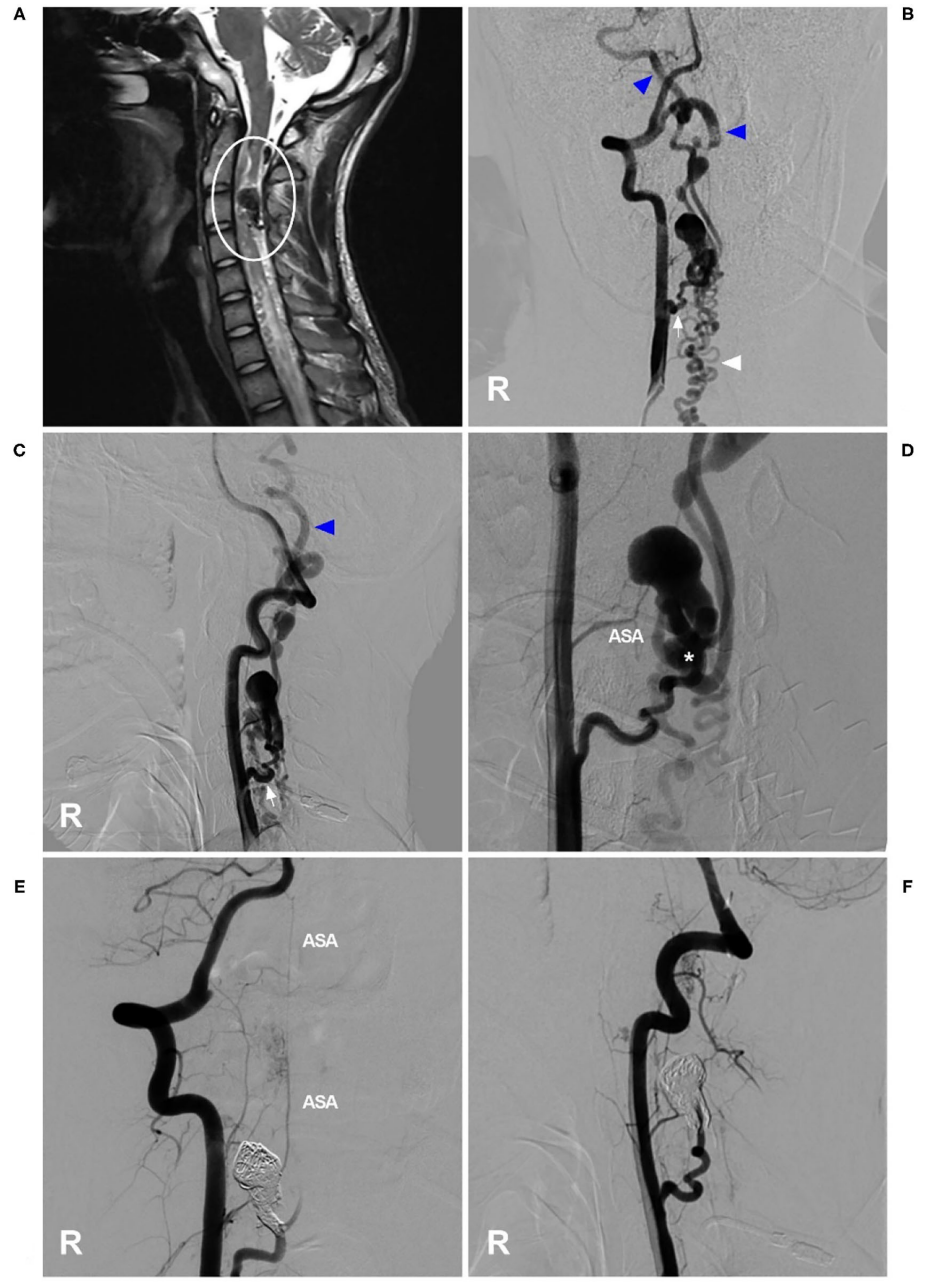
EVT of a cervical perimedullary arteriovenous fistula. (A) MRI showed a PAVF near the C3 level (circle). (B,C) Right VA anteroposterior view (B) and lateral view (C) angiograms showed that the PAVF was mainly supplied by a dilated radiculomeningeal artery (arrow), with draining to the cranium (blue triangles) and lower neck (white triangle), and that the draining veins were dilated. (D) An angiogram showed the shunt point of the PAVF (asterisk). The ASA could be seen as a bystander. (E,F) Right VA anteroposterior view (E) and lateral view (F) postoperative angiograms showed that the PAVF was embolized by coiling. The ASA remained intact as a bystander. ASA, anterior spinal artery; EVT, endovascular treatment; MRI, magnetic resonance imaging; PAVF, perimedullary arteriovenous fistula; R, right; VA, vertebral artery.
